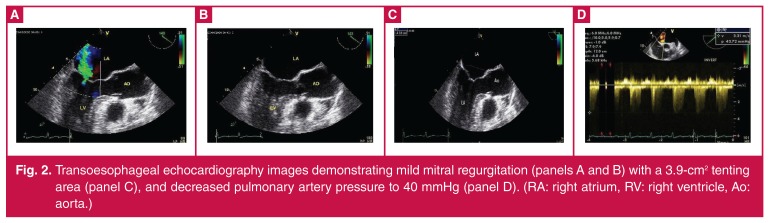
Transoesophageal echocardiography images demonstrating mild mitral regurgitation (panels A and B) with a 3.9-cm2 tenting area (panel C), and decreased pulmonary artery pressure to 40 mmHg (panel D). (RA: right atrium, RV: right ventricle, Ao: aorta.)
